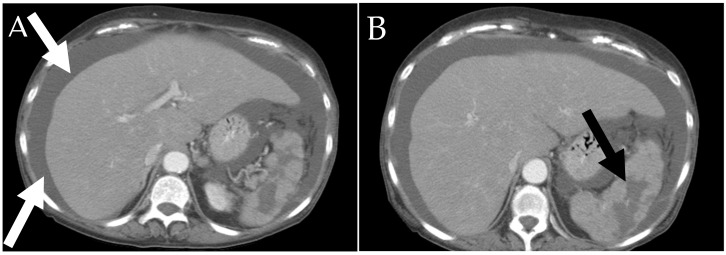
A 69-year-old female with history of systemic mastocytosis. (A,B) Axial contrast enhanced CT shows ascites (white arrows) which appears as free fluid at the liver margin. An enlarged heterogeneous spleen with lobulated contour and evindence of infarction (black arrow).
